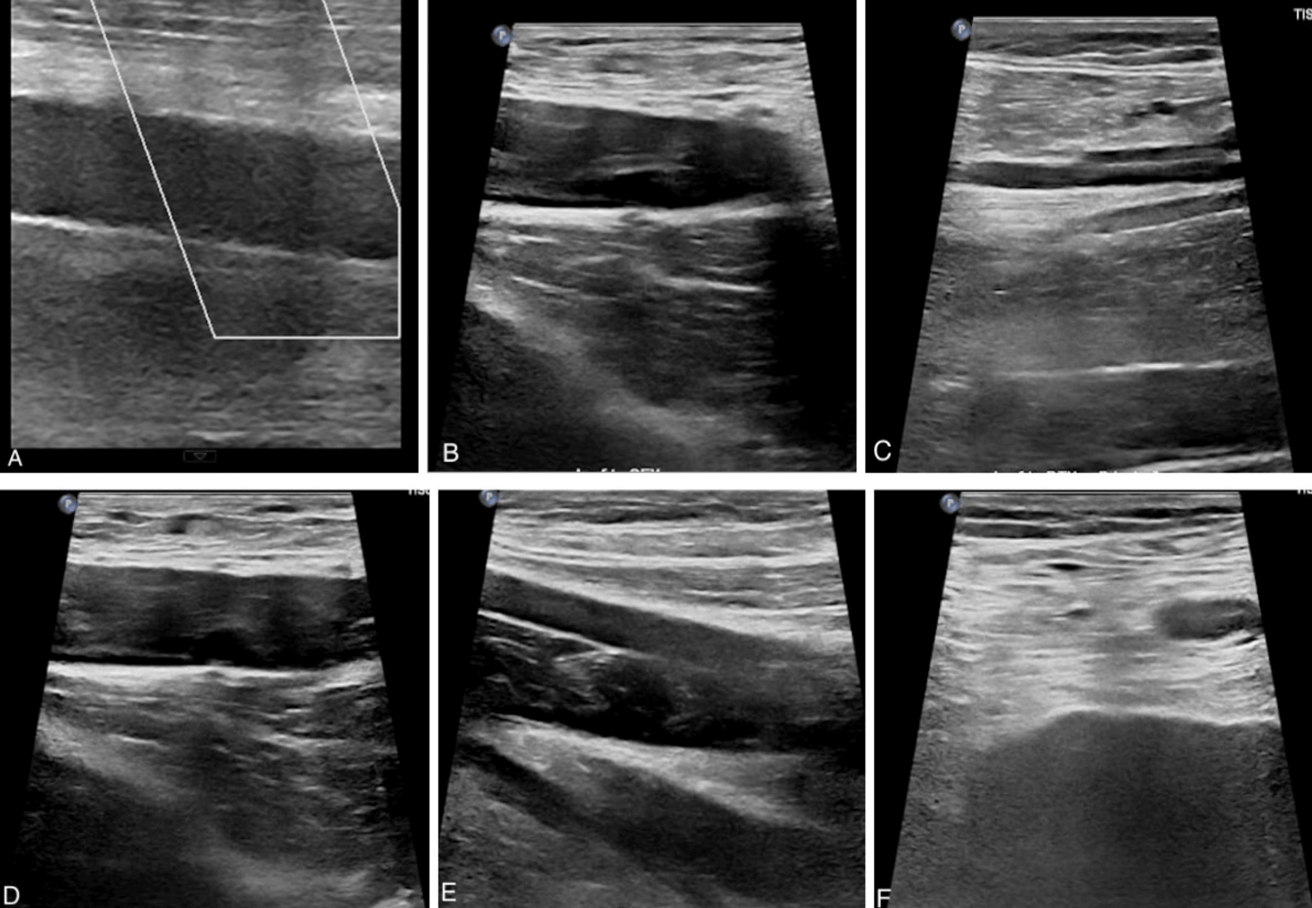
Venous ultrasound - greyscale images of the bilateral lower limbs veins showing echogenic thrombus (A) right common femoral vein; (B) right superficial femoral vein; (C) right popliteal vein; (D) left common femoral vein; (E) left superficial femoral vein; and (F) left popliteal vein.
